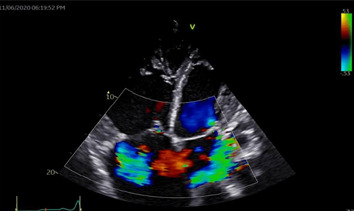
two-dimensional ECHO with colour doppler (apical four-chamber view) showing flow across the ASD and also severe mitral and tricuspid regurgitations
